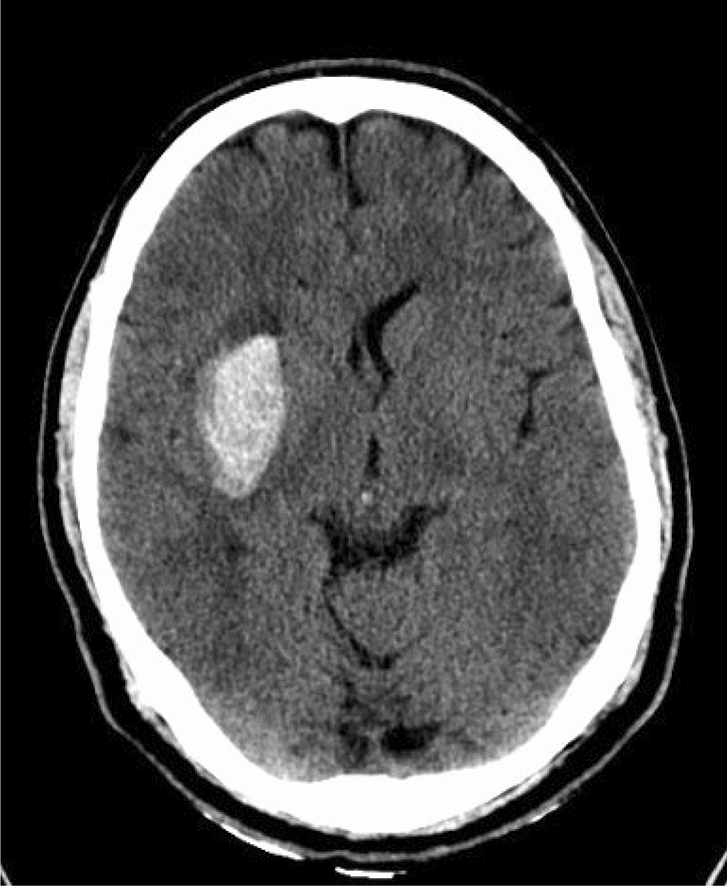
39-year-old man with history of hypertension. Axial non contrast CT scan shows right basal ganglia hemorrhage with surrounding edema. These findings are low probable of underlying vascular lesions and no vascular etiology was found on brain CTA.
